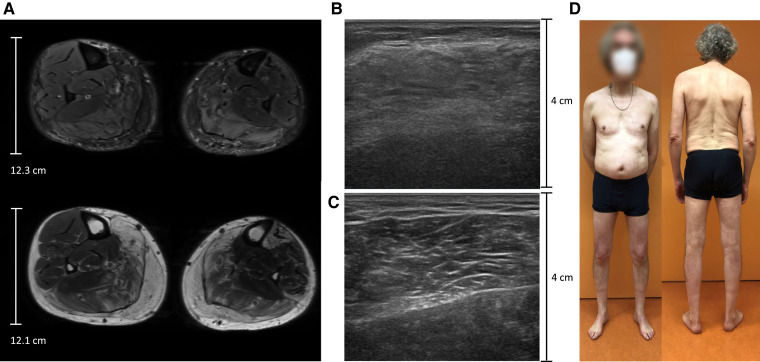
Magnetic resonance imaging, muscle ultrasound images and clinical photographs from study participants. (A) Magnetic resonance imaging Dixon T2 images and Dixon T1 images showing bilateral edema of the gastrocnemius and soleus muscle as well as fatty infiltration of the left gastrocnemius, tibialis anterior and semimembranosus muscles. The muscle magnetic resonance imaging and muscle ultrasound (B and C) were performed because of late-onset proximal muscle weakness. This patients carries the *RYR1* c.7300G > A, p.Gly2434Arg variant. (B) Muscle ultrasound imaging showing markedly increased echo intensity (Heckmatt rating scale^39^ 4) of the right medial gastrocnemius muscle This ultrasound image is from the same patient whose magnetic resonance images are shown in Fig. 4A and whose muscle ultrasound images are shown in Fig. 4C. (C) Muscle ultrasound images of the right lateral gastrocnemius muscle showing increased echo intensity (Heckmatt rating scale^39^ 2). This ultrasound image is from the same patient whose magnetic resonance images are shown in Fig. 4A and whose muscle ultrasound images are shown in Fig. 4B. (D) A study participant with late-onset proximal weakness and atrophy of the lower extremities as demonstrated in Videos 1 and 2. He carries the *RYR1* c.10616G > A, p.Arg3539His variant.
